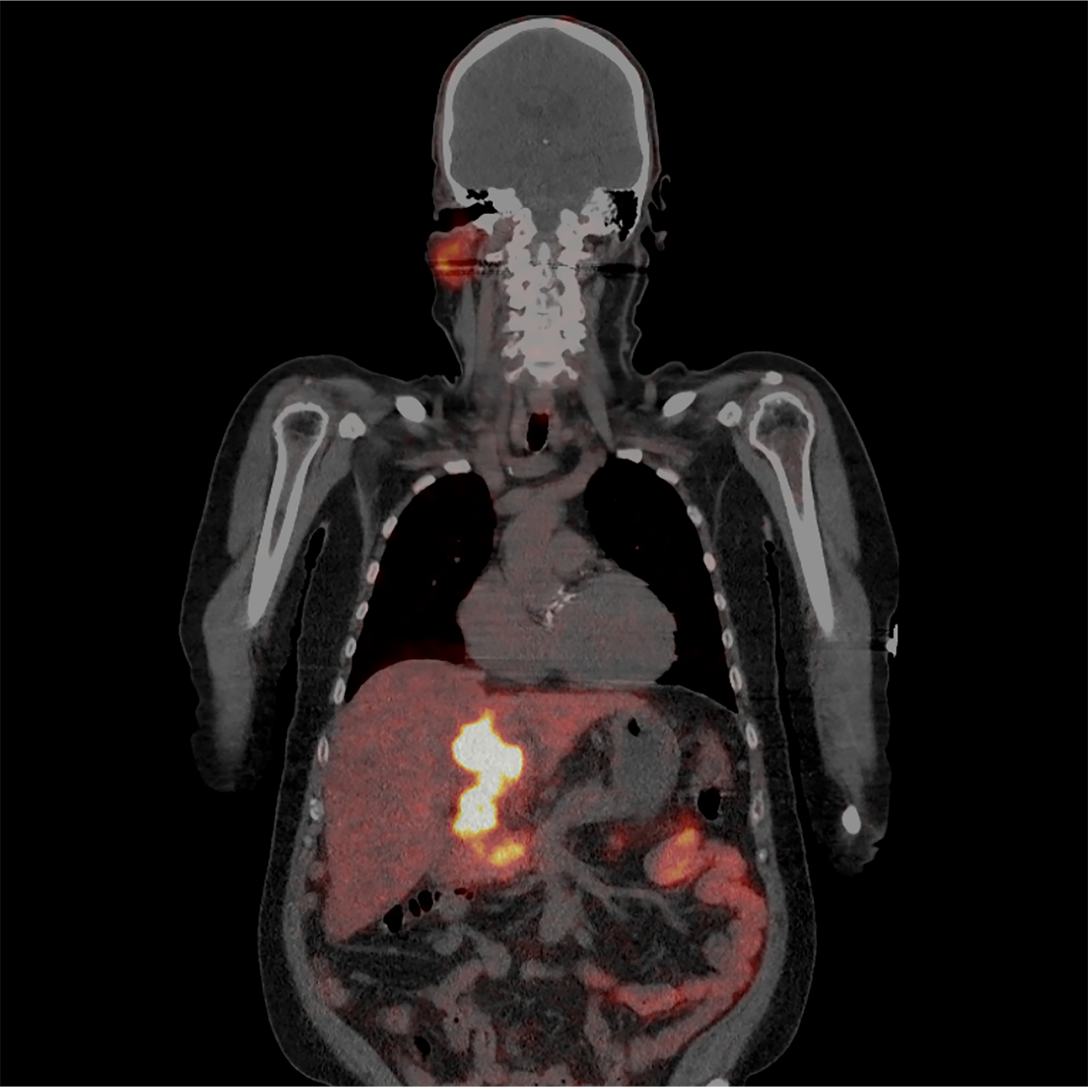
A 62-year-old patient with liver cirrhosis was diagnosed with HCC in 2015. He was primarily treated in December 2015 with radioembolization using ^90^Y-glass microspheres of segment 4 (300 Gy), November 2016 because of a progressive disease the patient received a second radioembolization of the left liver lobe. February 2017 due to further progress of the tumor he started with sorafenib. In August 2017 because of tumor progression a ^68^Ga-PSMA-11 PET/CT was performed to investigate the possibility of a radioligand therapy. Coronal view of the PET/CT scan shows an intense and heterogeneous PSMA uptake in HCCs.
